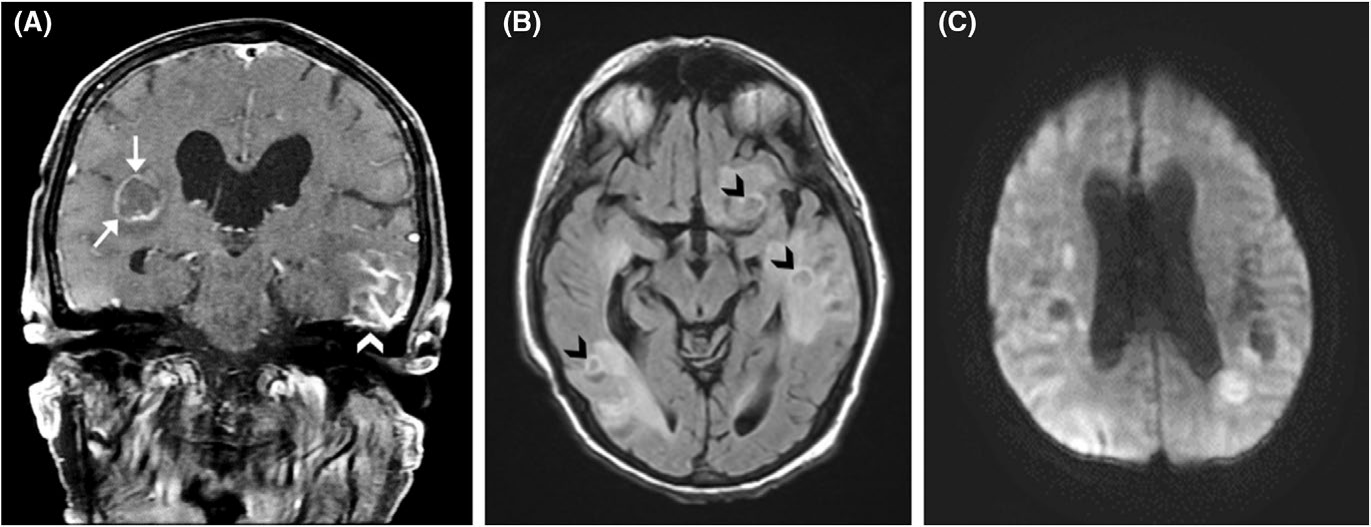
(A) Coronal post‐gadolinium T1‐weighted image demonstrates multifocal enhancement, including both ring‐enhancing lesions (white arrows) and more peripheral cortical involvement (white arrowhead). (B) Axial T2‐weighted FLAIR image displays vasogenic edema surrounding several centrally hypointense lesions (black arrowheads). (C) Axial diffusion weighted imaging (DWI) reveals that the lesions show varying degrees of diffusion restriction, in both solid and peripheral patterns
